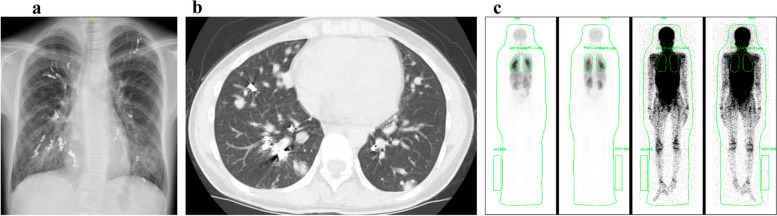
Chest X-ray radiography (a) and computed tomography (b) showing diffuse pulmonary arteriovenous malformations, which were treated with transcatheter coil embolization. Pulmonary perfusion scintigraphy showing an intrapulmonary shunting rate of 68% (c)
